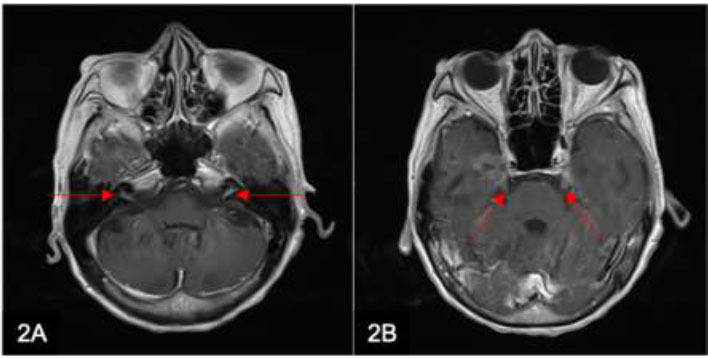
A 71-year-old woman presenting with partial seizures and a background history of lung cancer with brain metastases. Axial T1w postcontrast MRI brain images show enhancement of the bilateral 7th and 8th nerve complexes (A arrows) and bilateral 5th nerves (B arrows), consistent with leptomeningeal spread of the disease.
